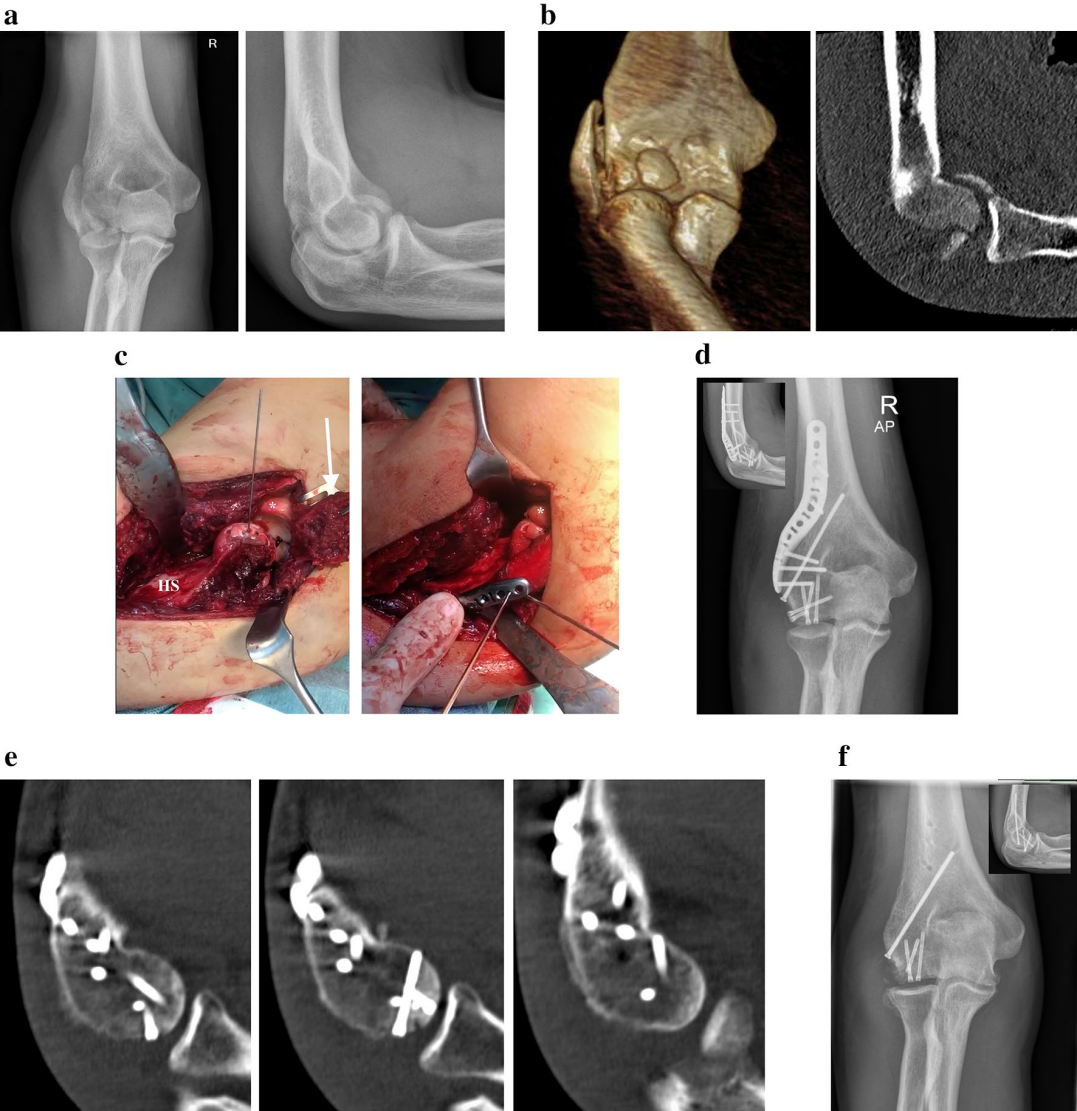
Case of a 32-year old male patient with a comminuted capitulum fracture (Dubberley type 2B) with concomitant radial condyle fracture of the right elbow. A Plain radiographs preoperatively. B Preoperative CT scan. C Intraoperative view. Following reflection of the radial condyle (white arrow), the articular surface can be restored. The radial condyle is then reduced and internal fixation with a precontoured lateral distal humerus locking plate is performed. D Plain radiographs postoperatively. E CT scan at 3 months to confirm anatomic reduction and fracture union. F Plain radiographs following partial implant removal and ligament bracing of the LUCL
